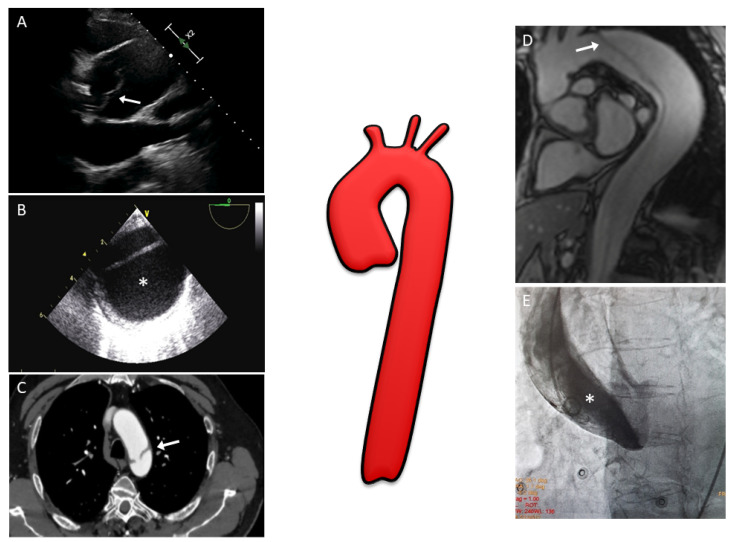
Multimodality imaging assessment of aortic dissection: (A) Two-dimensional transthoracic echocardiography showing a linear echo of an intimal flap (arrow) in a dilated aortic root above aortic valve level; (B) Two-dimensional transesophageal echocardiography in patients with aortic dissection involving the entire aorta; the false lumen (*) is typically larger and often compresses the true lumen, potentially affecting distal aortic flow; (C) CT image with evidence of the intimal tear (arrow) at the level of the aortic arch; (D) MRI with SSFP imaging in the oblique sagittal plane showing an intimal flap (arrow) from the aortic arch to abdominal aorta; (E) Aortic angiography performed in a patient with suspected inferior ST-segment elevation myocardial infarction revealing a type A aortic dissection (one may note that the pigtail catheter is located in the false lumen (*) of the dissection). CT, computed tomography; MRI, magnetic resonance imaging; SSFP, steady-state free precession.
